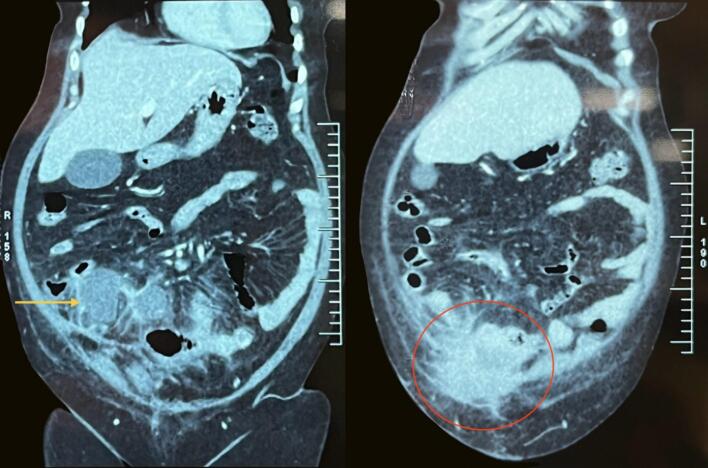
CECT abdomen and pelvis coronal section showing hyperechoic lesion in the right lower quadrant of the abdomen (yellow arrow) extending into the anterior abdominal wall (red circle). CECT: Contrast-Enhanced Computed Tomography. (For interpretation of the references to colour in this figure legend, the reader is referred to the web version of this article.)
